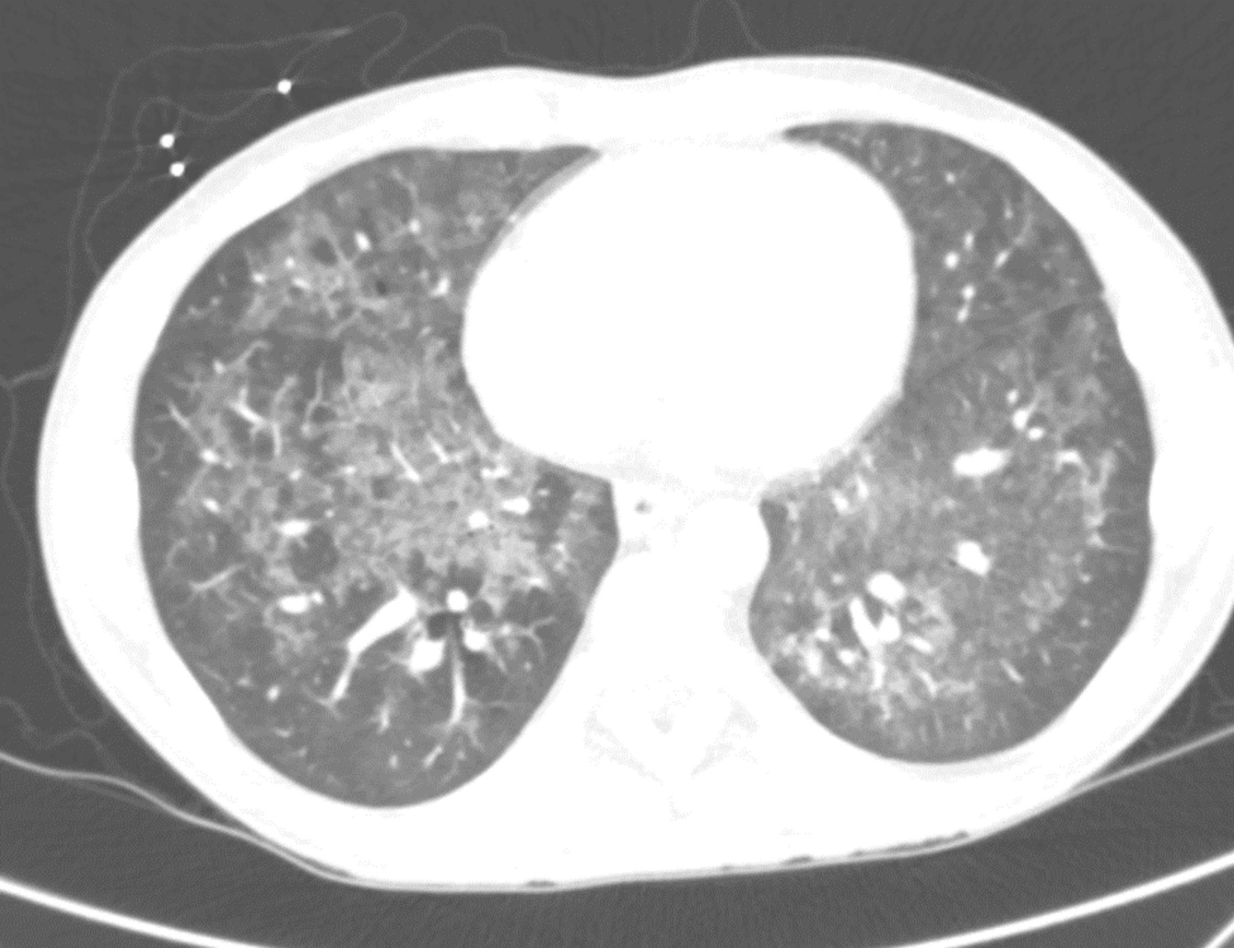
CT axial view demonstrating diffuse ground-glass opacities with peripheral sparing
